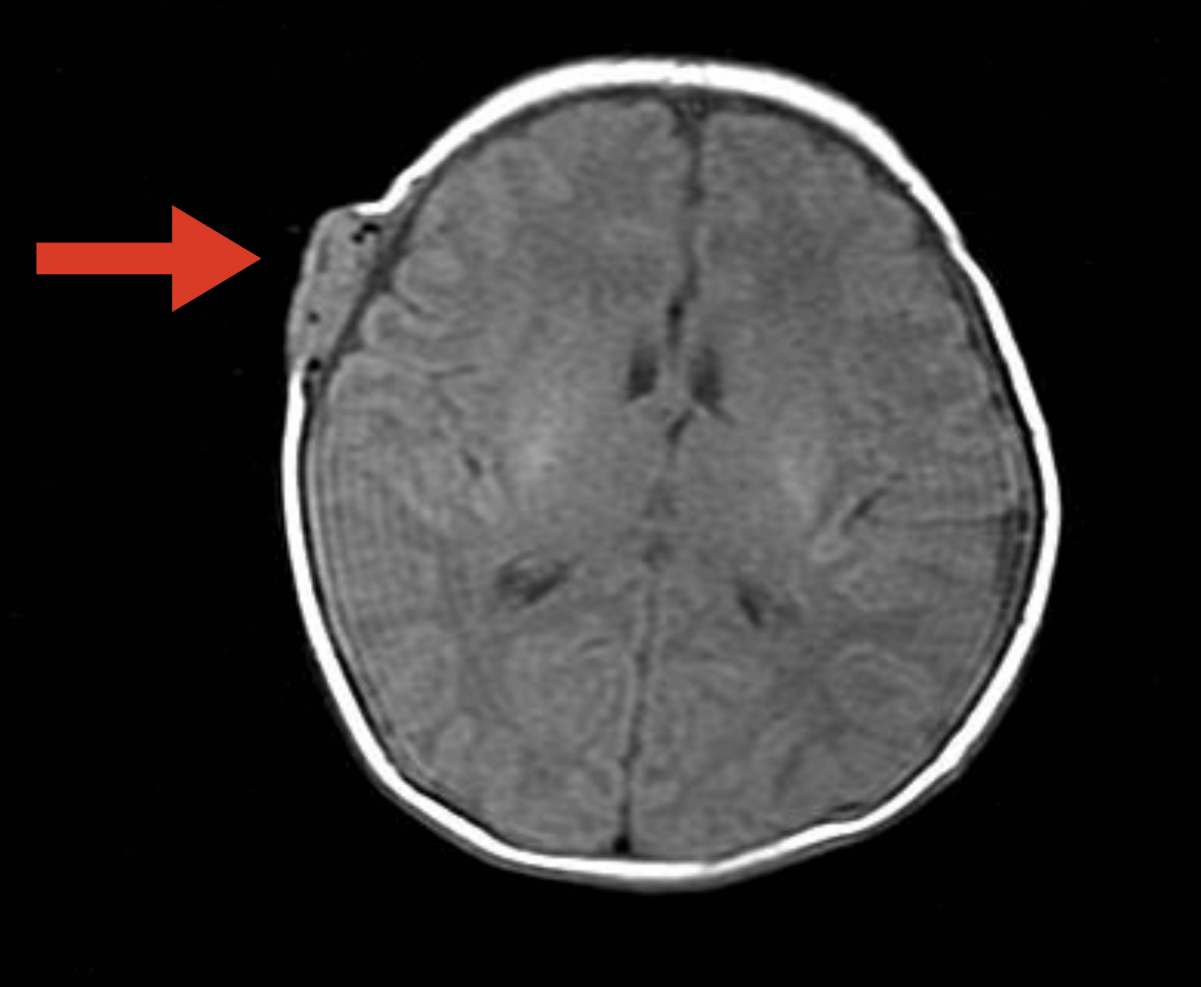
Magnetic resonance imaging with T1-weighted image showed the isosignal mass.
